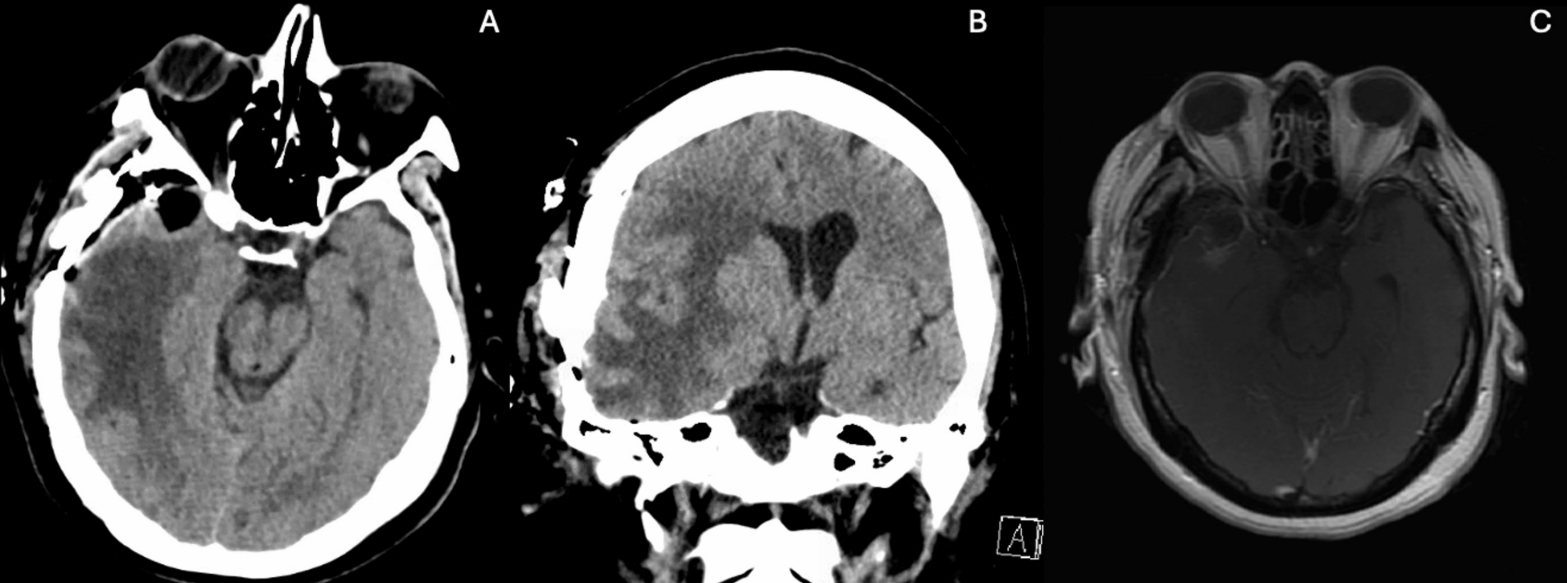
Postoperative images after intracranial meningioma resection. Immediate postoperative CT shows the surgical bed with the meningioma resected without complications (A), along with partial recovery of the midline and expansion of the previously collapsed right lateral ventricle (B). Contrast-enhanced MRI demonstrates gross total resection of the meningioma (C).
